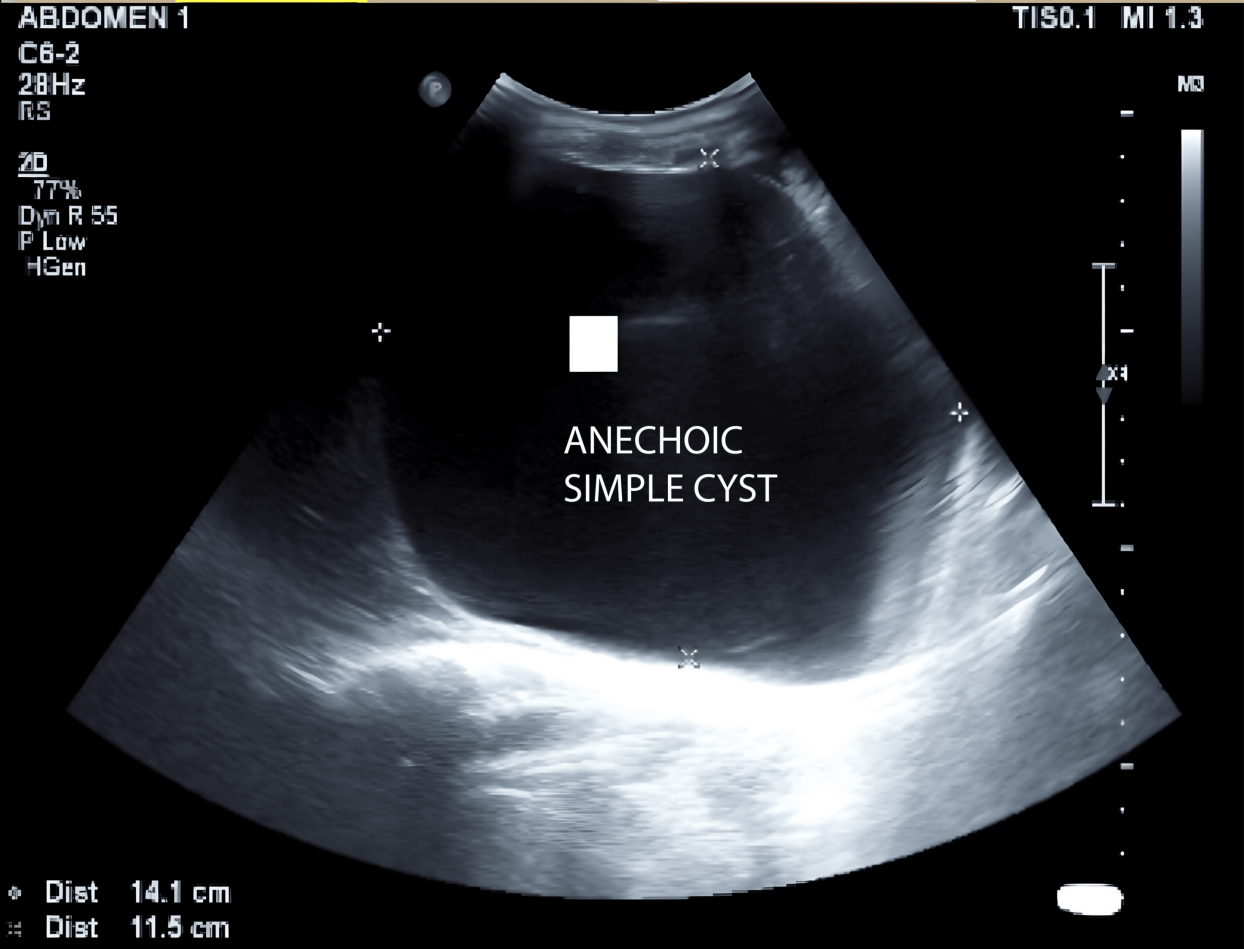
Ultrasound of the abdomen - image 1 A large anechoic cyst measuring 11.5 x 14 cm is seen in the right hepatic region. It has thin walls with no evidence of septations, solid components, or calcifications within
